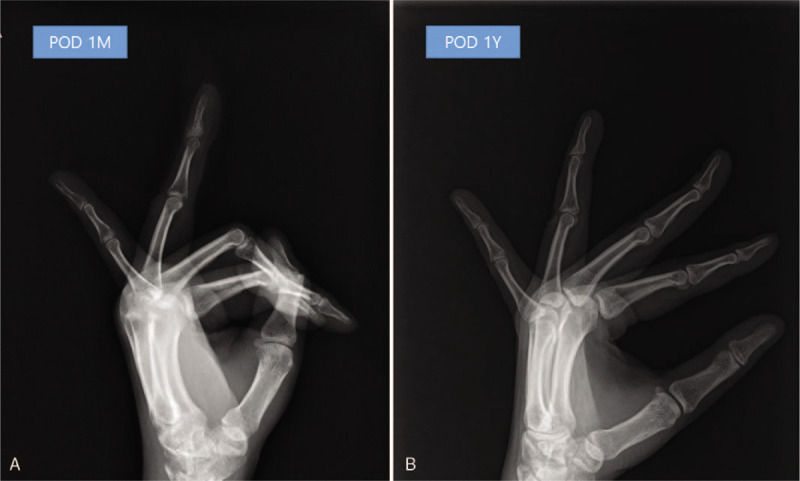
Radiographs showing postoperative results of curettage and bone grafting at 1 mo (left panel) and 1 yr (right panel). Full union of the pathologic fracture was evident at 1 yr postoperatively.
